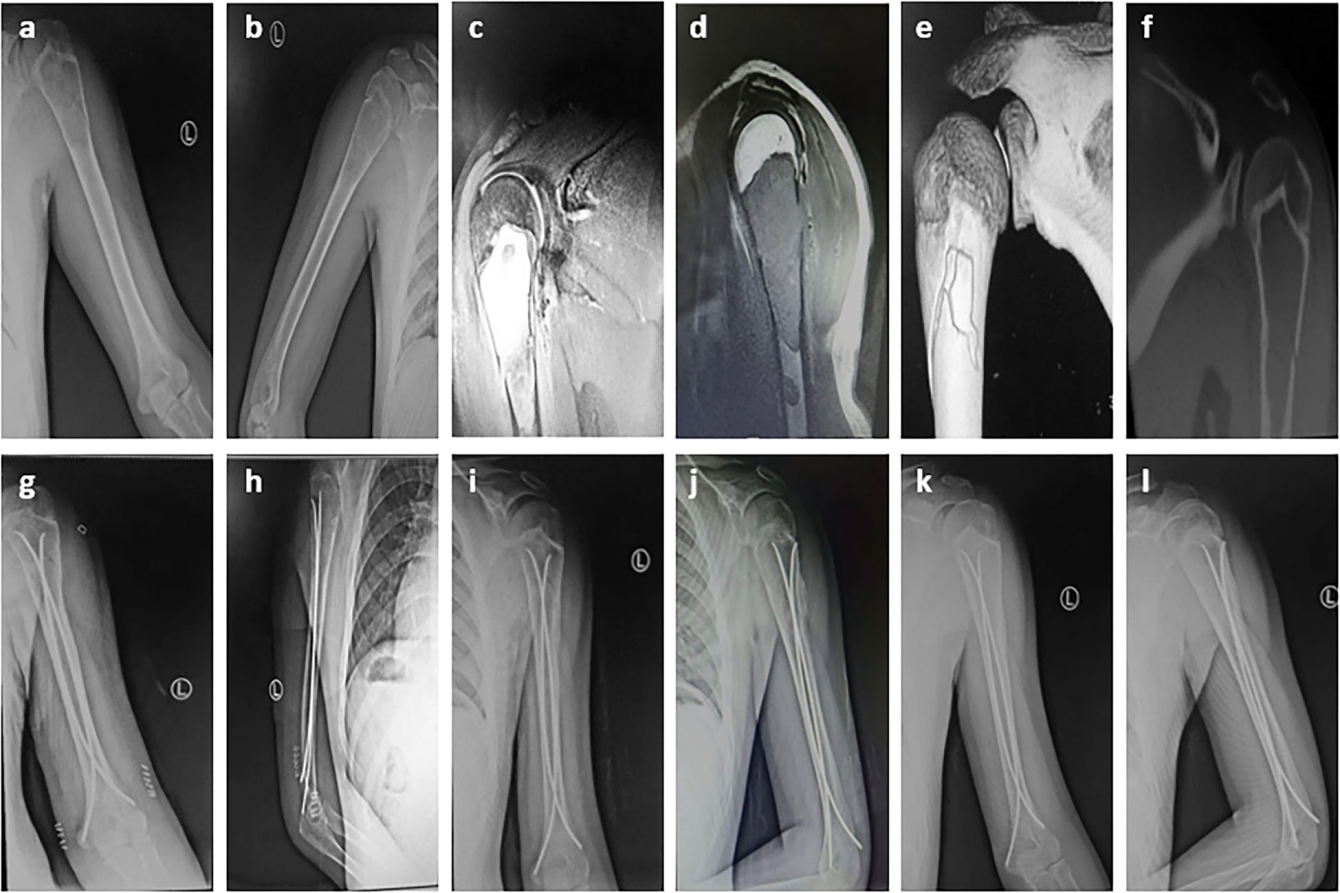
Radiographs of an 11-year-old boy who presented with pathological fracture of the left humerus. a-b Anteroposterior and lateral radiograph on admission. c-d MRI indicates a low signal on T1-weighted images and a homogeneous high signal on T2 weighting. e-f Three-dimensional CT image reconstruction and a sagittal CT scanning show fracture of proximal humeral bone cyst. g-l After the operation of minimally invasive intramedullary decompression and drainage with ESIN combined with intralesional injections of steroids; At the interval points of postoperative 1 weeks, 2 months, 6 months, the lesion is significantly smaller and shows signs of healing gradually, the fracture gradually healed, Radiographs show complete cyst healing (Capanna grade 1 healing)
